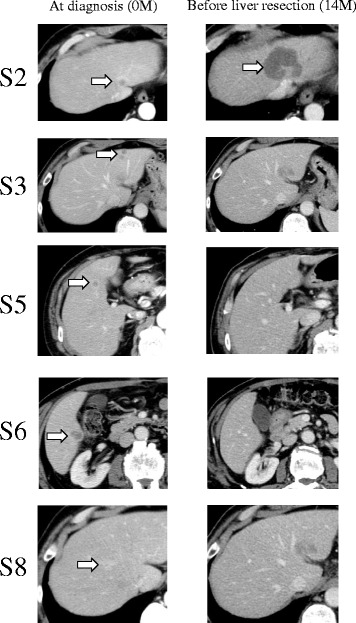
Computed tomography (CT) showed an enhanced mass in the rectum and hypo-vascularized tumors in the liver segment 2 (S2), segment 3 (S3), segment 5 (S5), segment 6 (S6), and segment 8 (S8). The largest liver tumor had a diameter of 16 mm in S2. Almost all of the multiple liver tumors had shrunk after the neoadjuvant chemotherapy, but the S2 tumor had grown on the CT scan (16 to 46 mm)
